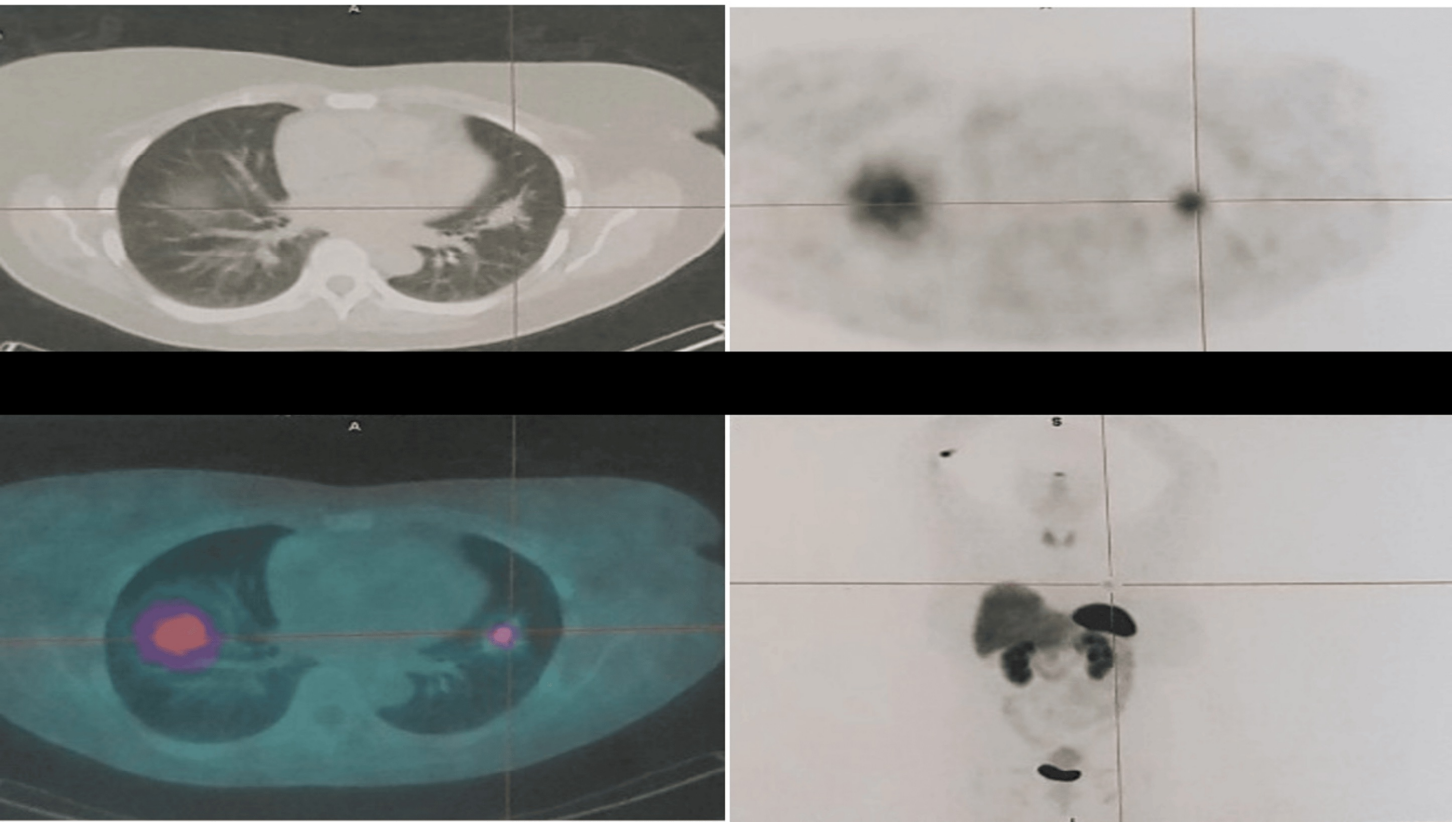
68Ga-DOTANOC PET/CT showing a single uptake in the lingula. Abbreviations: PET/CT, positron emission tomography-computed tomography
